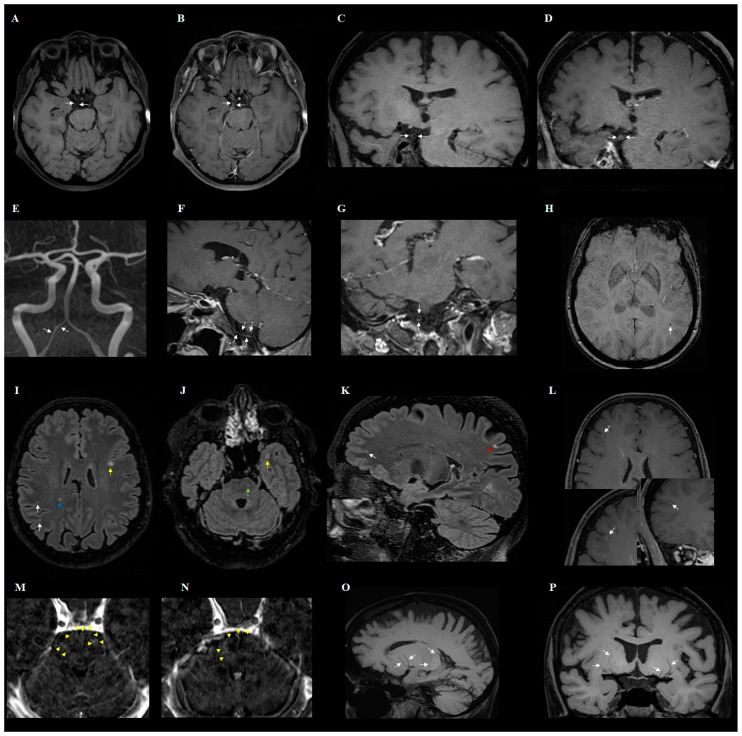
Magnetic resonance imaging of the brains of different patients with post-COVID-19 conditions. (A) Axial and (C) coronal sagittal pre-contrast 3D T1-weighted images, (B) contrast-enhanced axial, and (D) coronal sagittal 3D T1-weighted images show segmental concentric wall thickening and enhancement of the right fetal posterior cerebral artery (arrows), consistent with cerebral vasculopathy. (E) Coronal maximum intensity projection (MIP) reconstruction of the 3D TOF MR angiography demonstrates mild segmental narrowing of the right vertebral artery (arrows) with corresponding vascular contour irregularity, concentric thickening, and enhancement of vessel wall on the (F) sagittal and (G) coronal post-contrast 3D T1-weighted images (arrows). (H) SWI image shows a cerebral microhemorrhage in the subcortical white matter (arrow). (I,J) Axial and (K) sagittal 3D-FLAIR images demonstrate several hyperintensities with varying distributions—subcortical (white arrows), juxtacortical (yellow arrows), cortical (red arrow), and within the deep white matter (blue arrow) and pons (green arrow). (L) Axial and coronal post-contrast 3D T1-weighted images show small foci of cortical contrast enhancement in the right frontal lobe (arrows). (M,N) Axial contrast-enhanced 3D T1-weighted subtraction maps at the level of the pons demonstrate signal abnormalities along the course of perforating vessels (arrowheads). (O) Sagittal and (P) coronal minimum-intensity projection (MinIP) reconstruction of the pre-contrast 3D T1-weighted images at the level of the basal ganglia show bilateral tortuosity and mild dilatation of the perforator vessels (white arrows).
